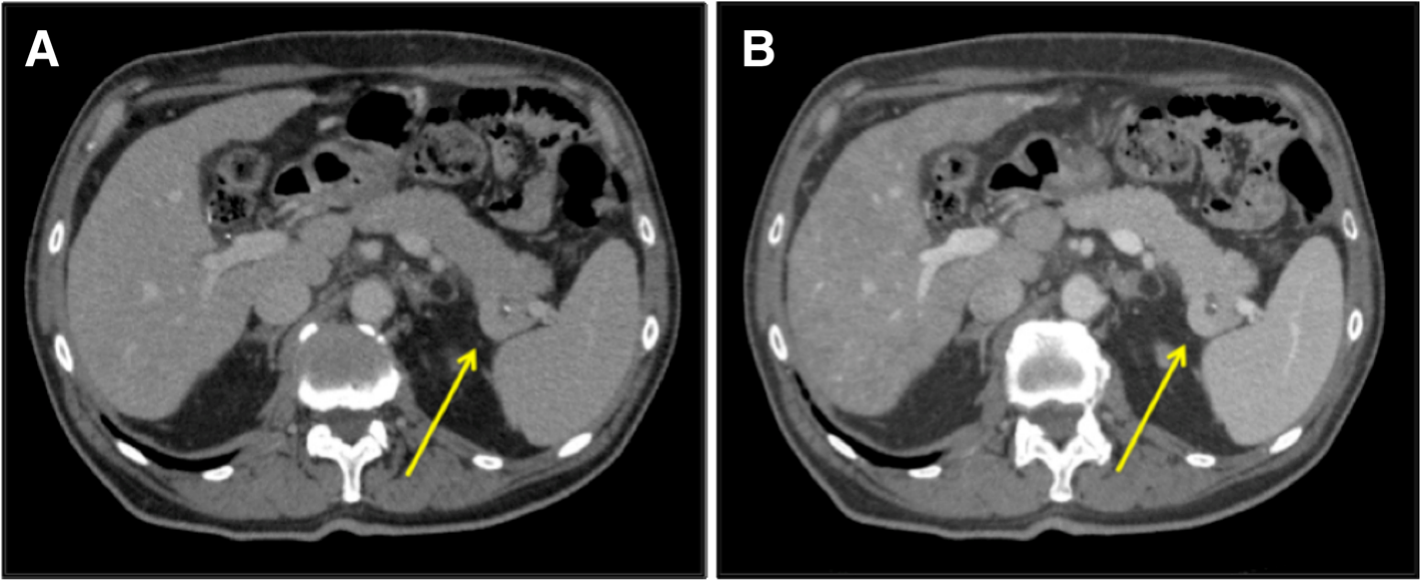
Incidental mass in the tail of the pancreas (arrow). CT revealed a mass well delimited with homogeneous contrast enhancement. a Venous and b arterial phase axial CT images
